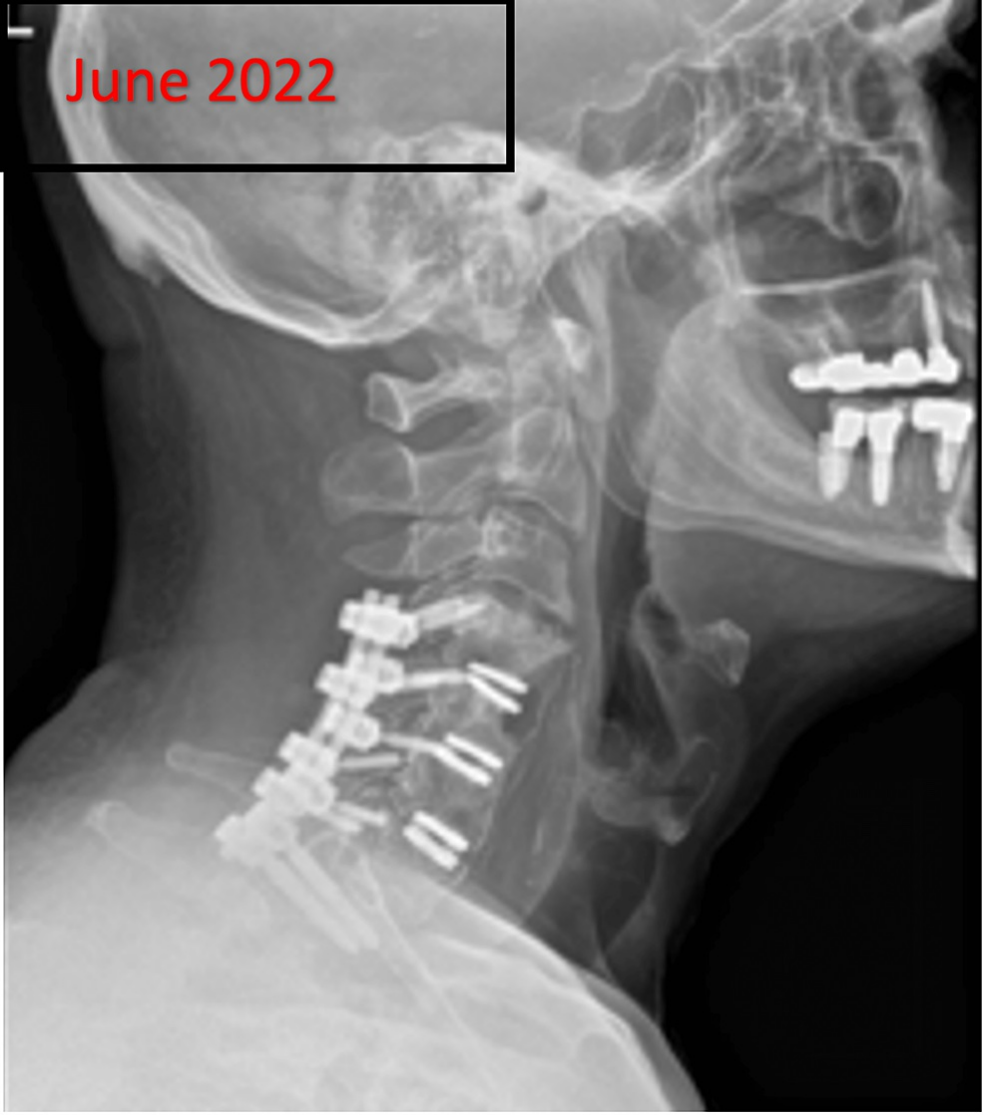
X-ray: Post-operative-Anterior discectomy at C4-C5, C5-C6 and C6-C7. The visualized soft tissues reveal no abnormalities.
